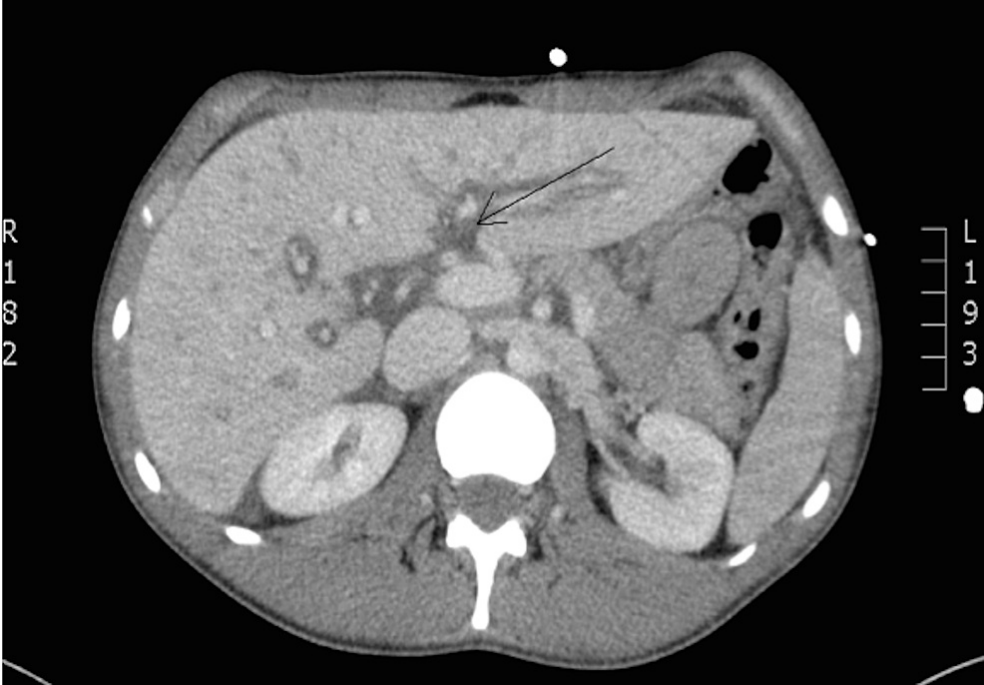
CT Abdomen and Pelvis with IV contrast demonstrating peri-portal edema. (black arrow)
